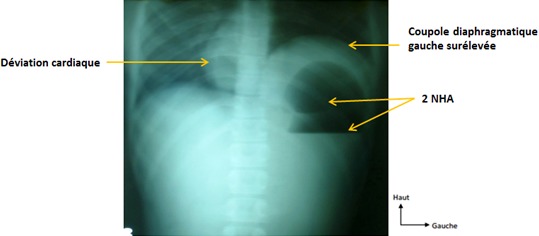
Radiographie de l'abdomen sans préparation
